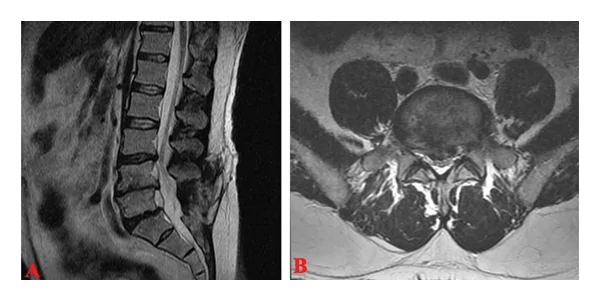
Two‐year postoperative magnetic resonance imaging (MRI) demonstrates resolution of the cyst with vertebral body changes consistent with discitis (A). The L5–S1 intervertebral space shows normal recovery (B).
